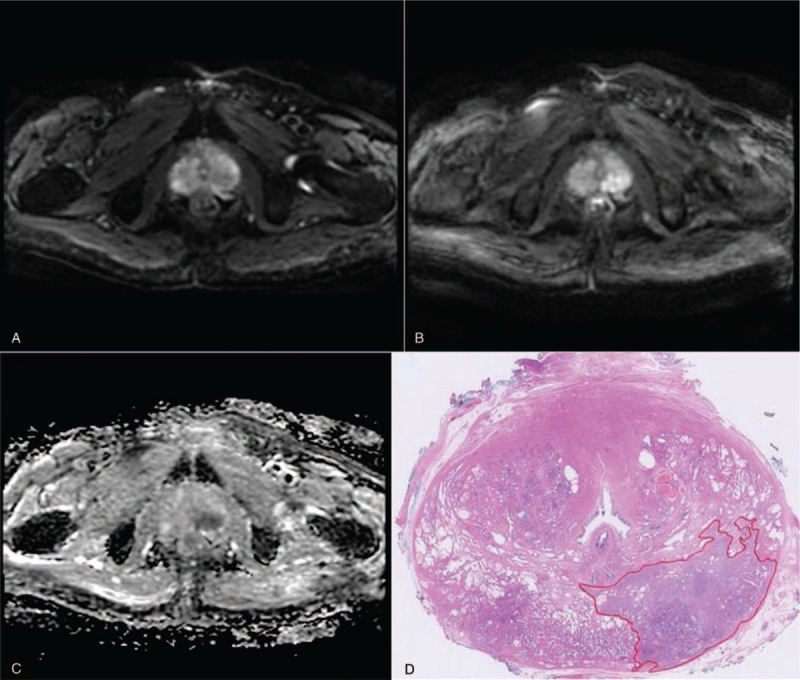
Results for a 72 years old man with prostate specific antigen of 8.7 ng/mL. Magnetic resonance imaging (MRI) suggested left posterior prostate cancer focus visible on (A) T2-weighted image, (B) diffusion weighted imaging, and (C) apparent diffusion coefficient map, the PI-RADS score was 5. A 3-core magnetic resonance-guided prostate biopsy and 11-region template-guided transperineal prostate saturation biopsy revealed Gleason 4 + 3 prostate cancer in left posterior prostate. The patient was treated with radical prostatectomy. (D) Whole-mount prostate section confirmed pT2 Gleason 4 + 3 prostate cancer. The tumor location corresponded to the location of positive cores and MRI. MRI = magnetic resonance imaging, PI-RADS = Prostate Imaging Reporting and Data System.
